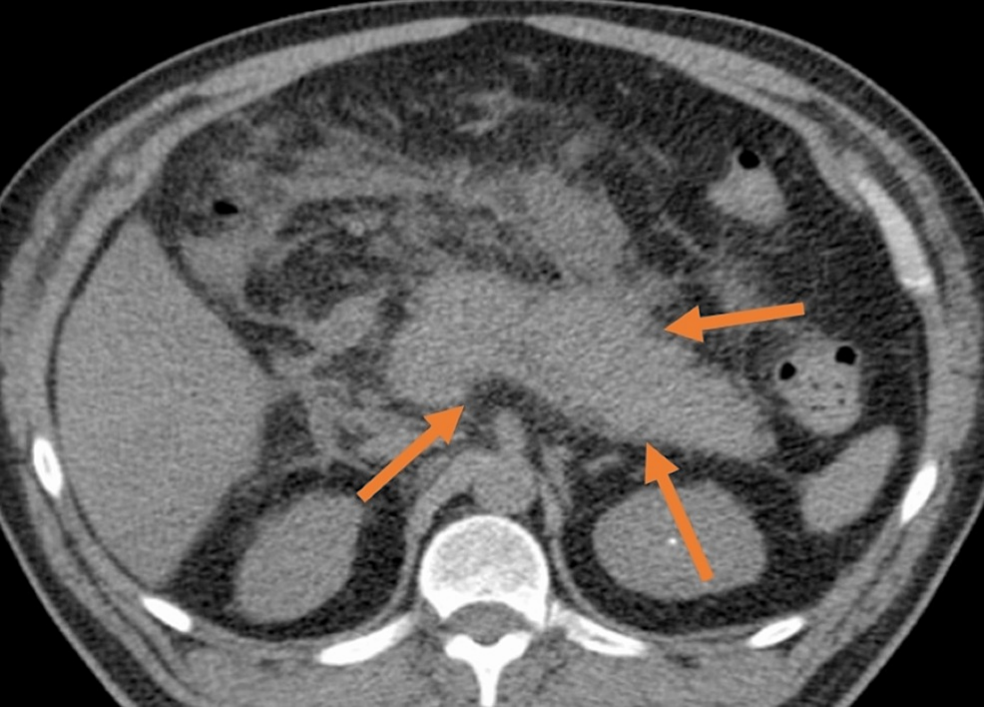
Abdominal computed tomography revealing diffuse pancreatic inflammation with ill-defined borders.
